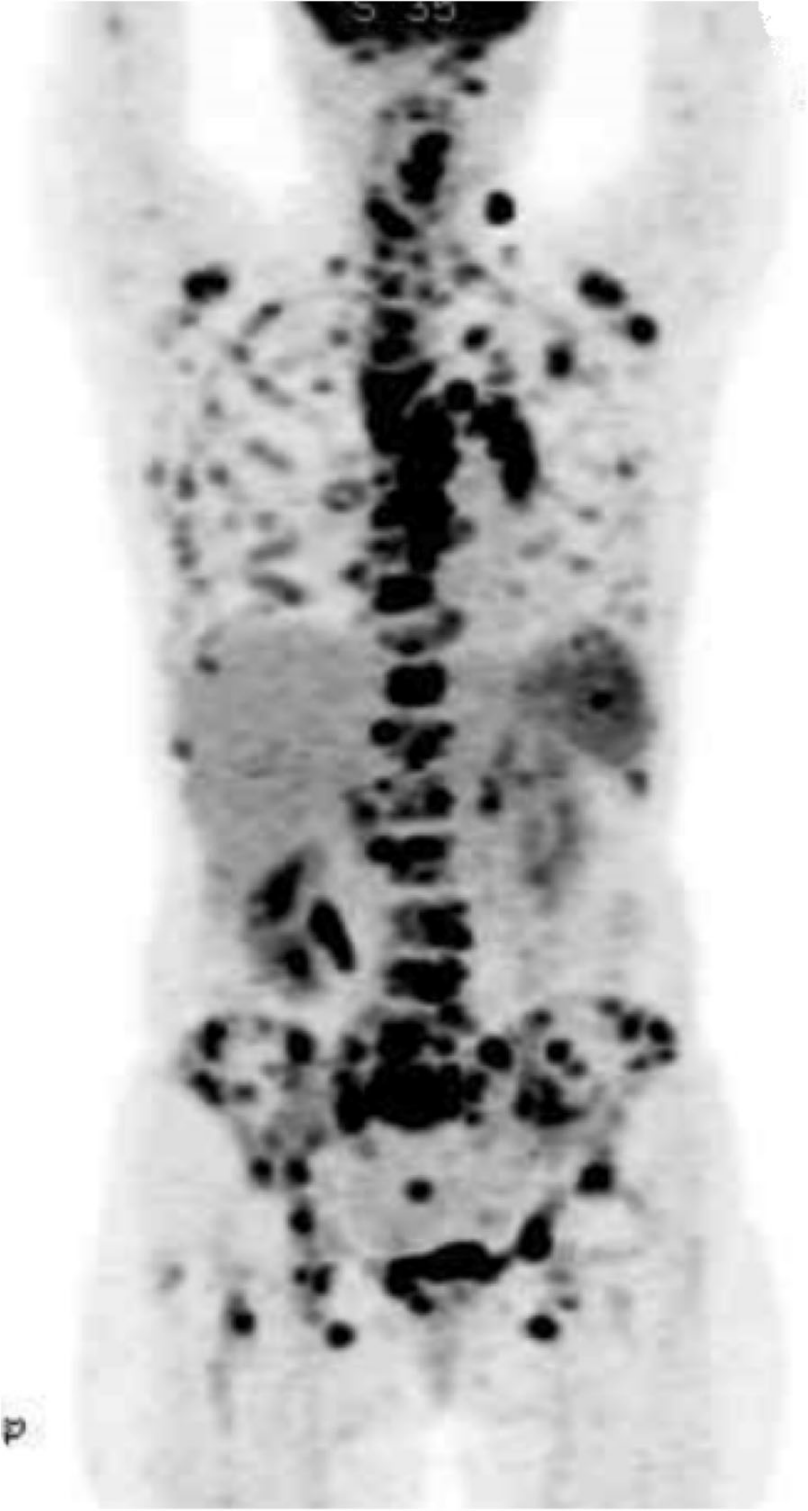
A maximum intensity projection image following fluorodeoxyglucose-positron emission tomography (FDG-PET) revealed multifocal hypermetabolic lesions in the nodule in the left S1 + 2 segment, interlobular septal thickening in the left lower lobe, lymphadenopathy of the left hilar, mediastinal, supraclavicular, and posterior cervical lymph nodes. FDG also accumulated in the anterior spinal cord, sacrum, iliac bone, pubic bone, ischium, sternum, scapula, ribs, clavicle, and thigh bone
